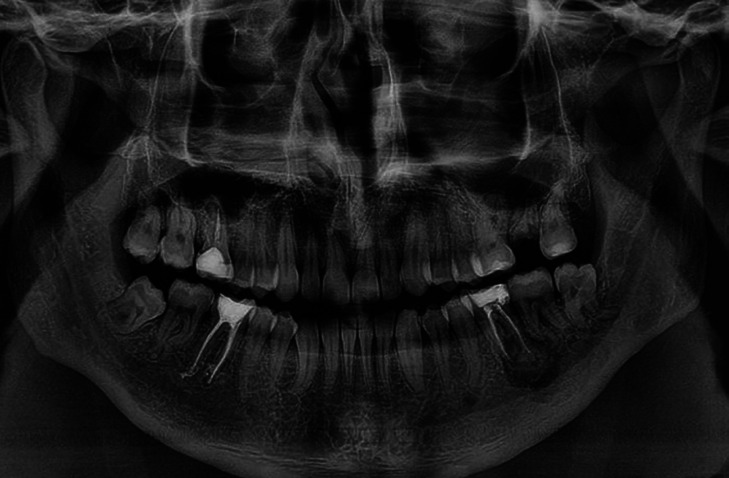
A panoramic radiograph shows the endodontically treated left mandibular first molar presenting a rounded radiopaque mass surrounded by radiolucency area attached to root.
